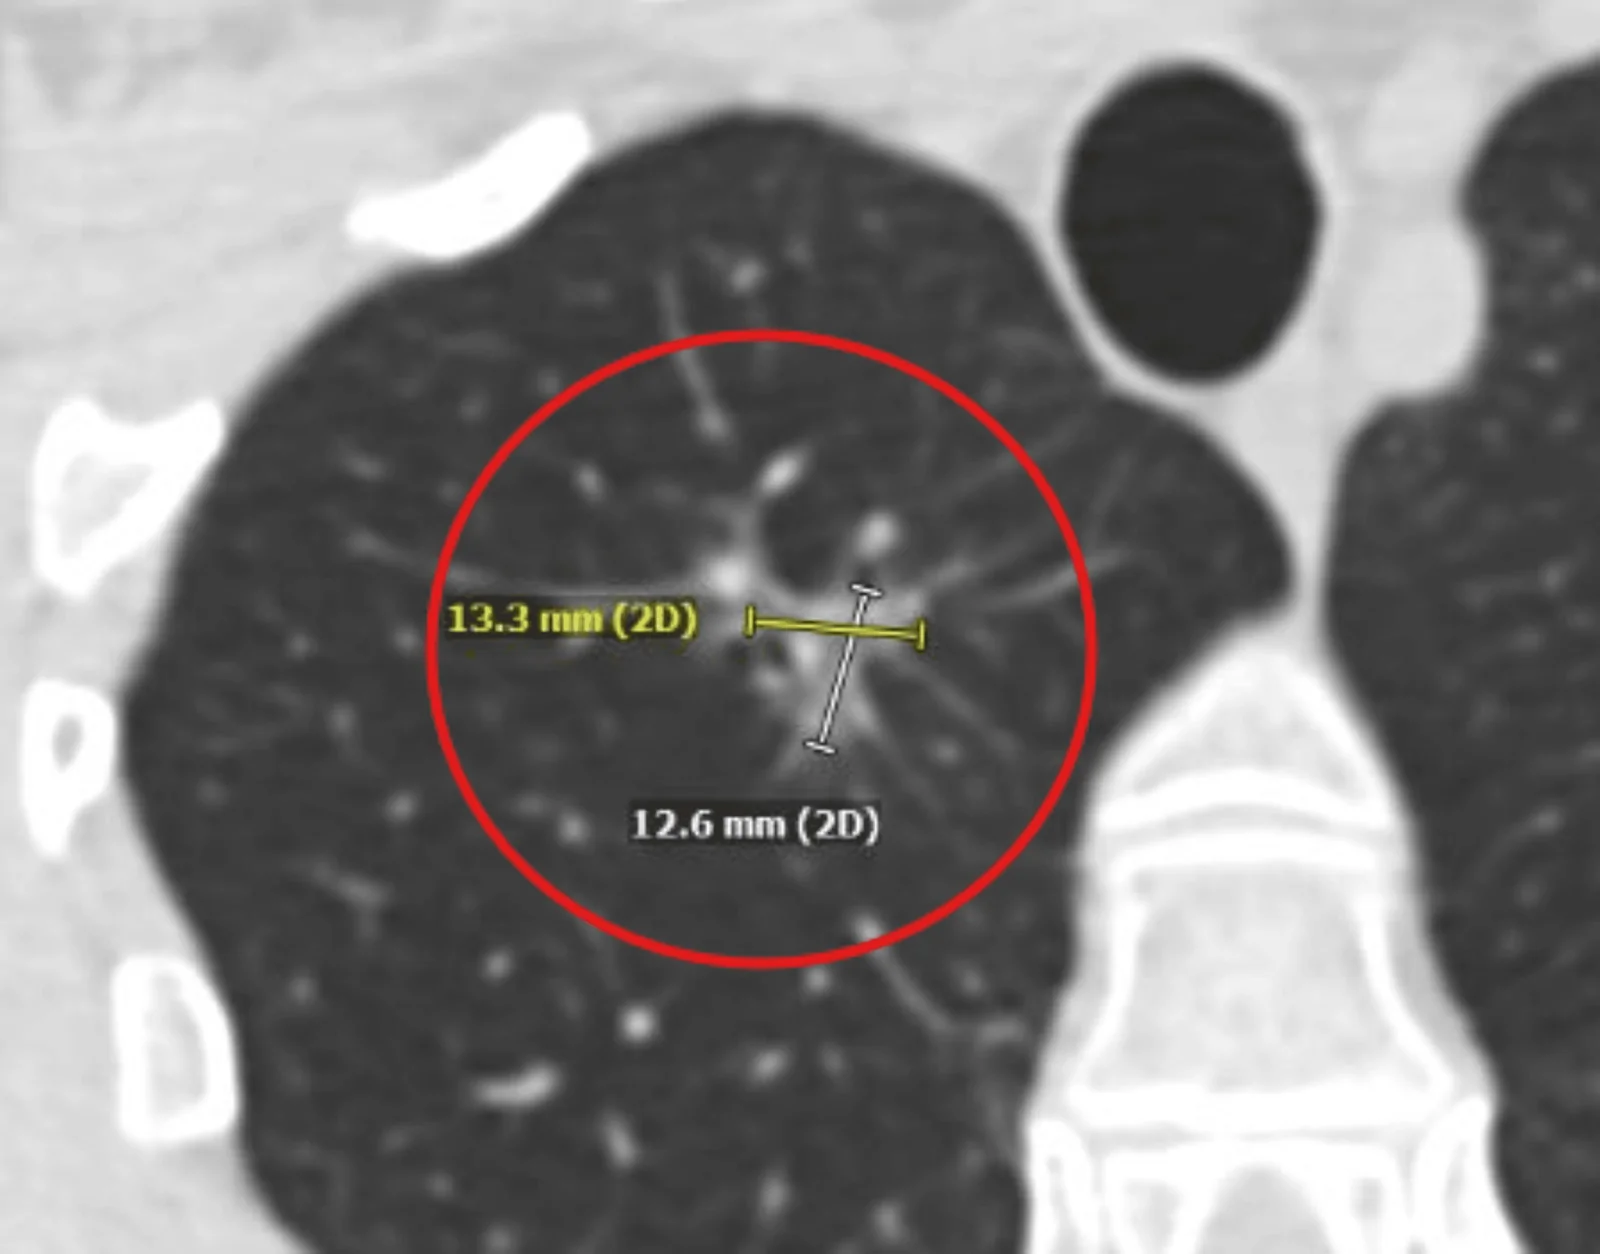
Selected axial non-contrast chest CT image obtained approximately seven years after the initial diagnosis, demonstrating interval enlargement of the right upper lobe subsolid pulmonary nodule with development of an approximately 8-mm solid component.
